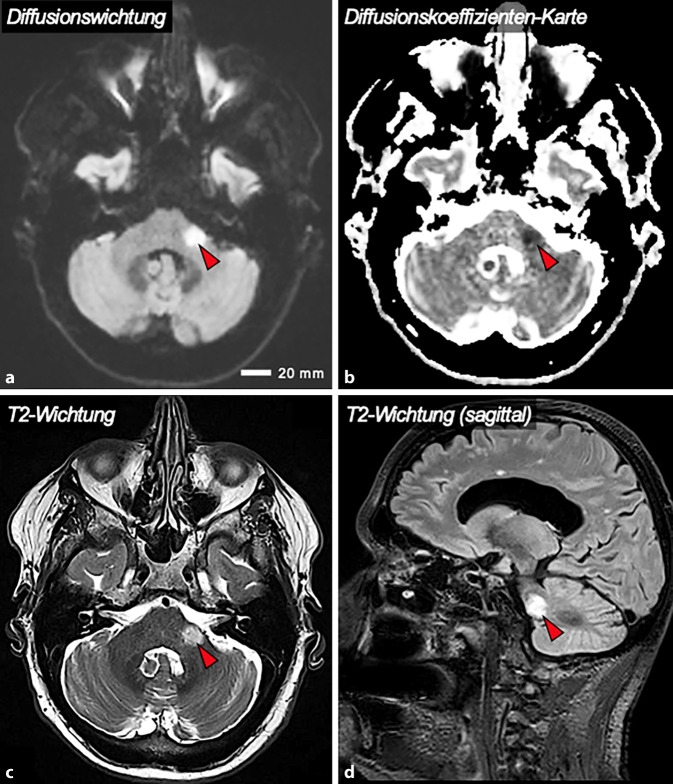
Bildgebende Diagnostik mittels Magnetresonanztomographie. Diese zeigte eine sphärische, diffusionsgestörte Läsion (*rote Pfeilspitze*) des linksseitigen Hirnstamms (a–c) und Kleinhirnstiels (d) unter Miteinbeziehung der Wurzeleintrittszone des Nervus vestibulocochlearis links. MR-Sequenzen: a Diffusionswichtung („Diffusion Weighted Imaging“, DWI); b Diffusionskoeffizienten-Karte („Apparent Diffusion Coefficient Map“, ADC-Map); c T2-Wichtung; d T2-Wichtung, „Fluid-Attenuation-Inversion-Recovery“(FLAIR)-Sequenz
